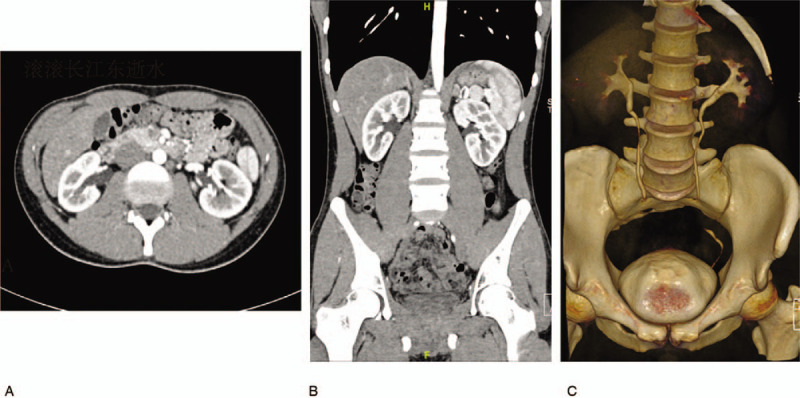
The CT urography showed bilateral extrarenal pelvis, and no other abnormalities were found in the kidneys, ureter, bladder, and vertebral bodies.
